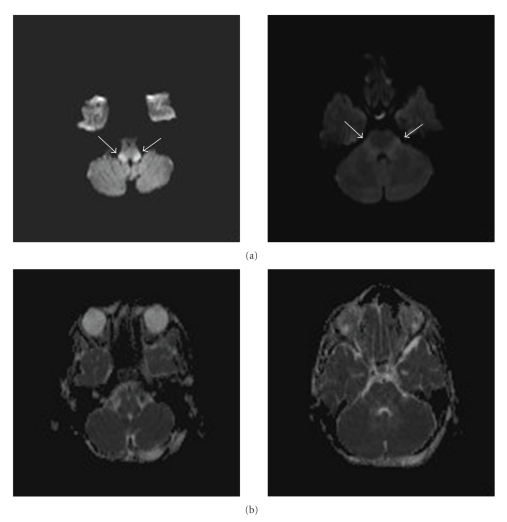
(a) Axial diffusion-weighted MR images show marked hyperintensity in some areas of the inferior and middle cerebellar peduncles. (b) On the apparent diffusion coefficient (ADC) maps, they are distinctly hypointense.
